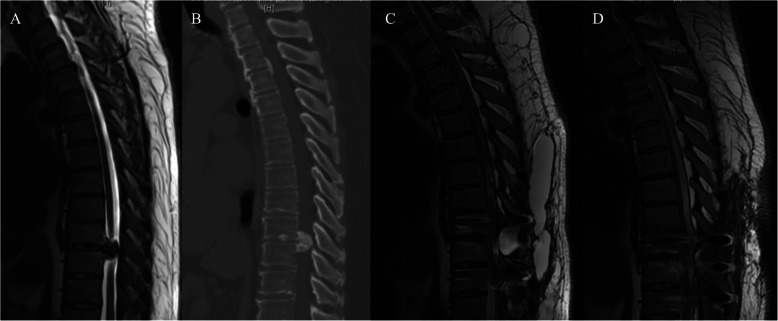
a, b Preoperative MRI and CT reveal that T10-11 OPLL and spinal cord compression. He received circumferential decompression of T10-11, with instrumentation from T10 to T12. He discharged at 4th postoperative day. Unplanned readmission occurred at 17th postoperative day due to local bulge under the incision, c MRI reveal CSF around the decompression region and subcutaneous pseudomeningocele. d He received revision surgery of tight suturing of the muscle and fascia layers, postoperative MRI revealed that the pseudomeningocele was cleared
